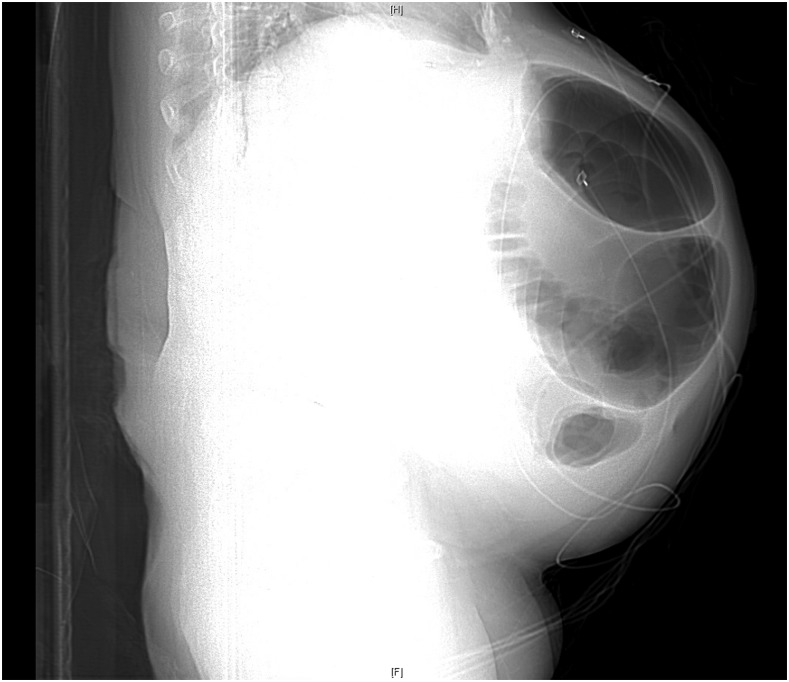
Scout view of the abdomen (lateral projection) on computed tomographic study of the abdomen and pelvis.
